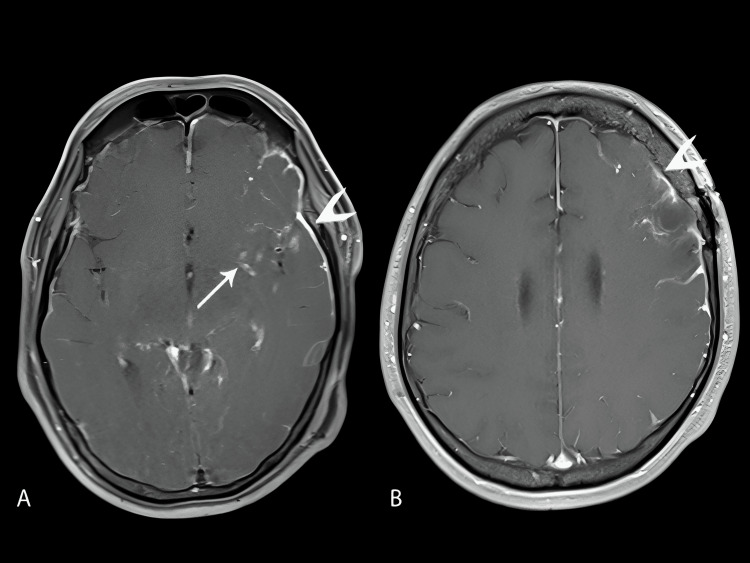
Additional MRI findings on day 48 Axial post-contrast T1-weighted images with fat saturation (A) and without fat saturation (B). The arrow shows abnormal enhancement of the perivascular spaces in the left basal ganglia (A), while the arrowhead highlights abnormal leptomeningeal enhancement along the left frontal lobe (A, B). A burr hole craniotomy and post-biopsy parenchymal defect are visible in the left middle frontal gyrus (B).
